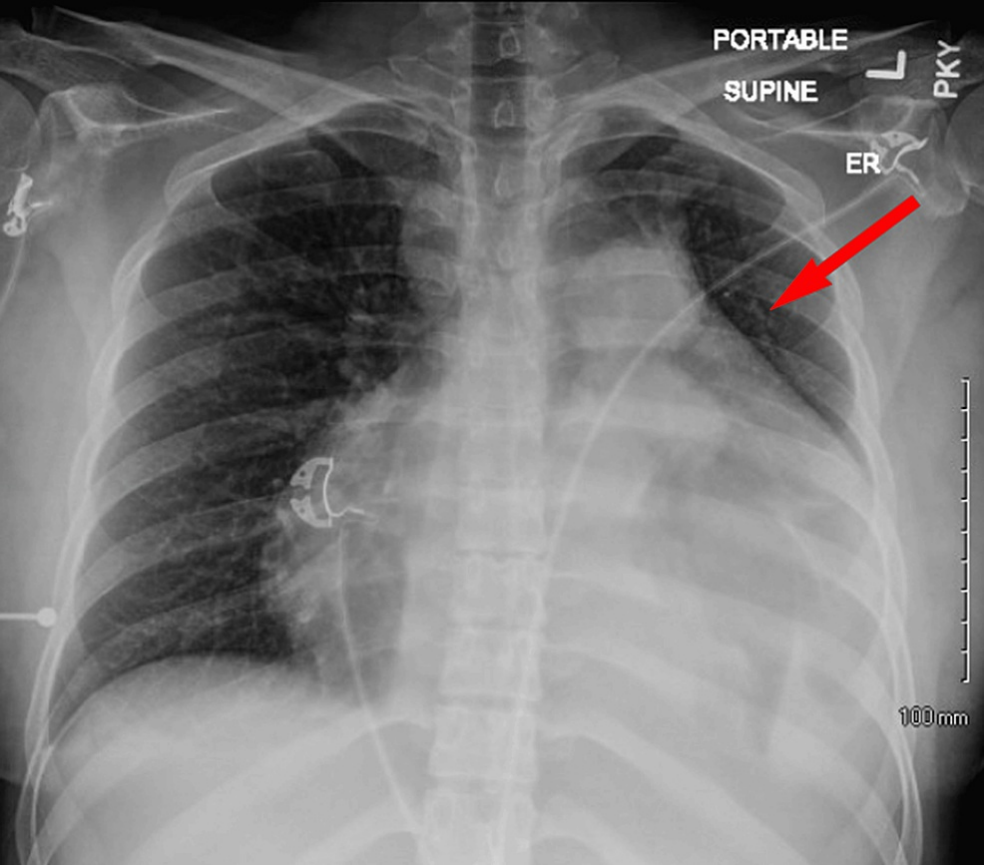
Chest x-ray on admission Cardiomegaly was noted with left retrocardiac opacity.
